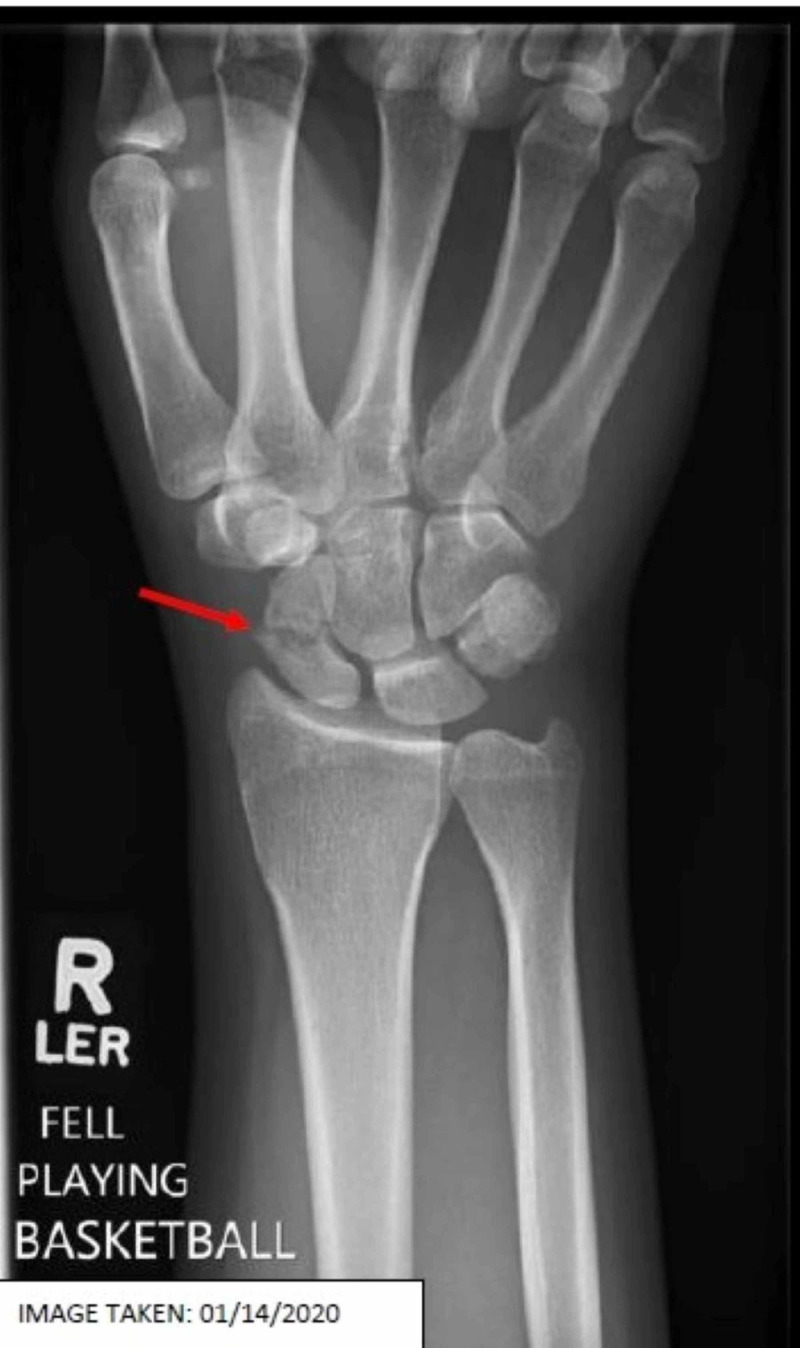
X-ray revealed scaphoid waist fracture with mild displacement, but overall good alignment, minimal callus, and incomplete healing (Red arrow)
